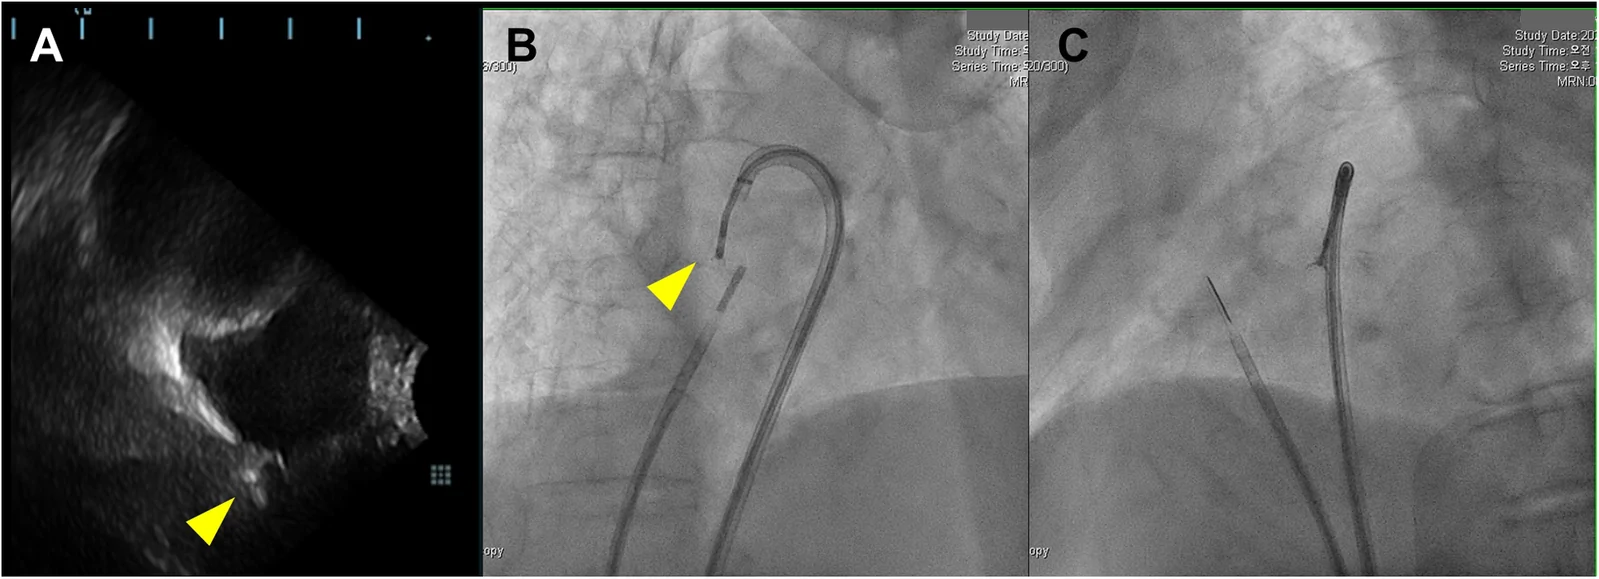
Intracardiac echocardiography guided left atrium forcep biopsy. (A) The biopsy forcep is visible (yellow arrow head). (B) and (C) The fluoroscopic right anterior oblique and left anterior oblique image during the biopsy using a deflectable sheath.
